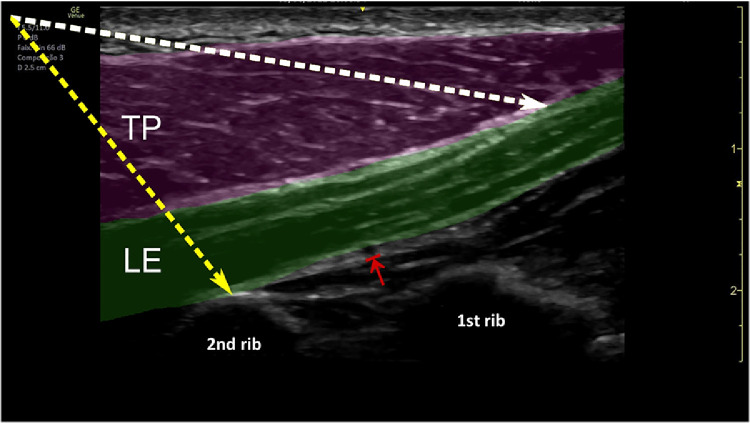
Levator scapular plane (LeSP) block in ultrasound view. TP, trapezius muscle in pink; LE, levator scapulae muscle in green; red arrow, dorsal artery of the scapula; white dashed line - LeSP 1 infiltration site; yellow dashed line - LeSP 2 infiltration site.
